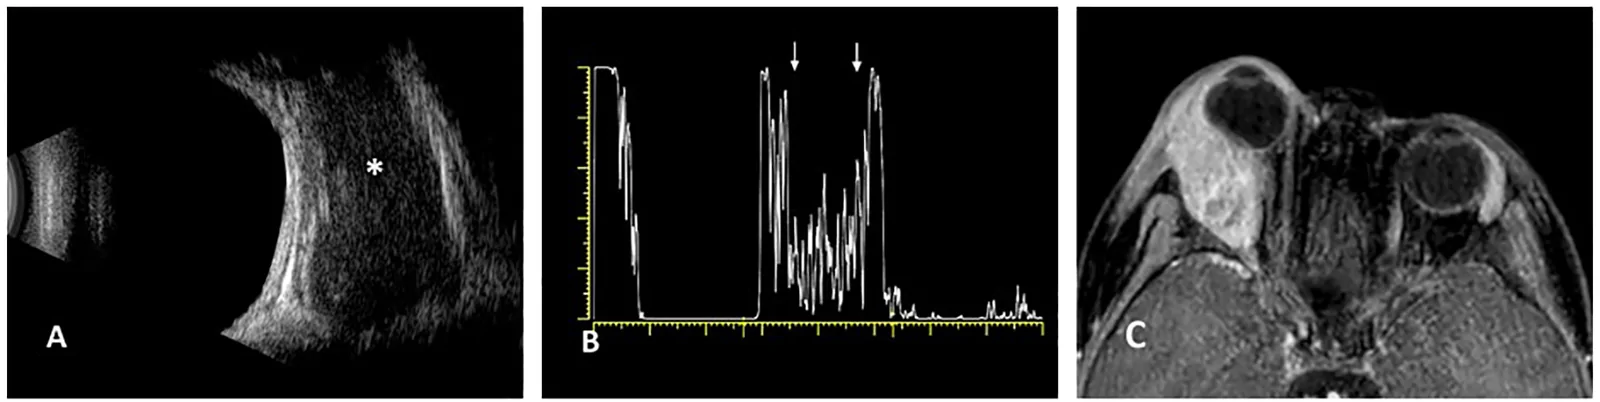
Orbital Rhabdomyosarcoma. A 9-year-old boy presented with a 4-week history of a right-sided orbital lesion. (A) Transverse B-scan shows a large, low reflective orbital mass (asterisk). (B) Corresponding A-scan shows low-amplitude internal spikes (arrows). (C) Axial T1-weighted MRI shows a contrast enhancement mass in the right orbit displacing the eye. The asterisk (*) is used to label an area of the figure.
